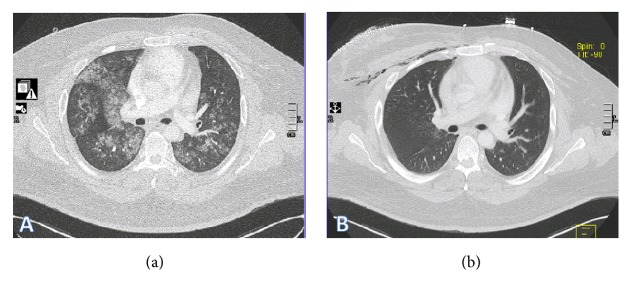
(a) Chest CT scan on admission with diffuse ground glass opacity and bilateral patchy consolidation. (b) Chest CT scan two weeks after admission showing improvement of parenchymal lesions. Patient developed mild subcutaneous emphysema following right lung wedge resection biopsy.
